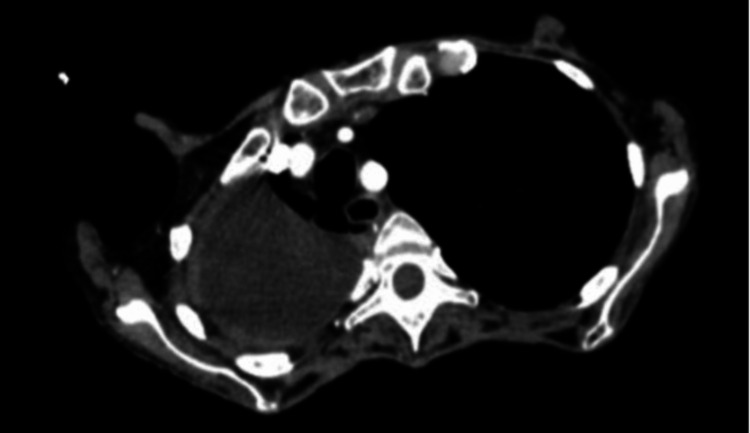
CT chest imaging demonstrating a loculated right upper lobe pleural effusion, necessitating further pulmonological testing
